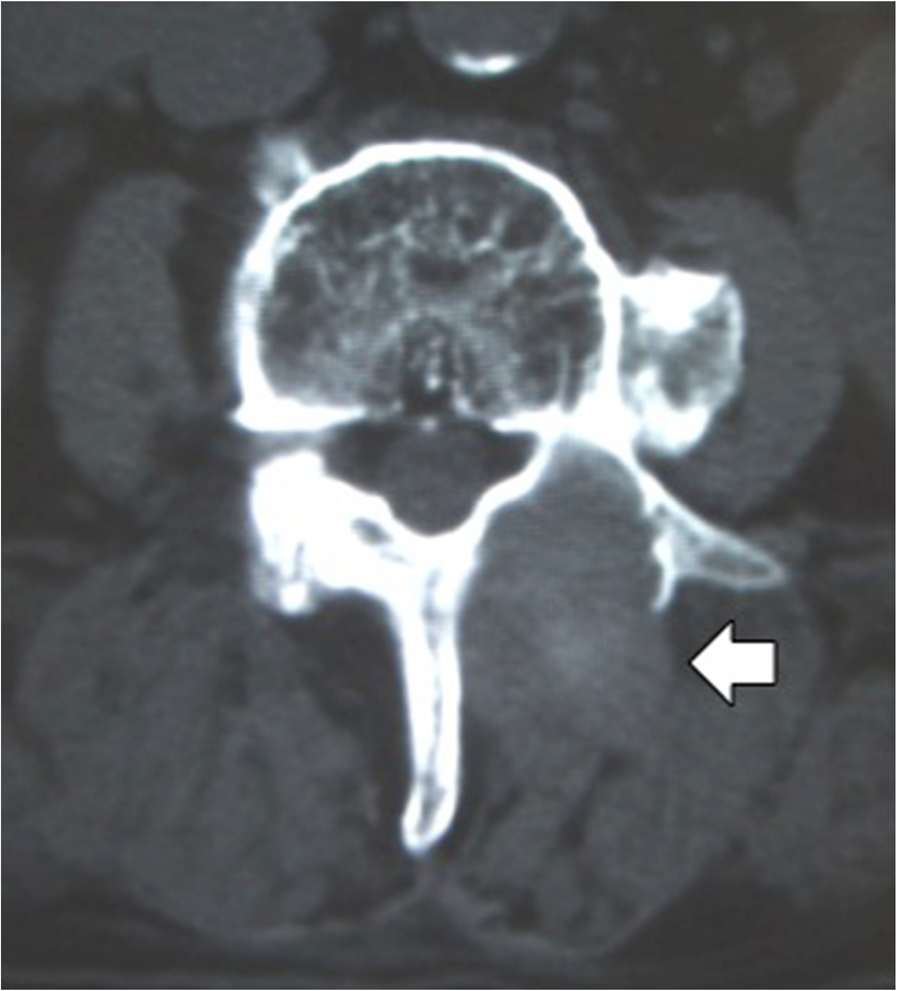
Computed tomography axial image. A mass (open arrow) is seen posterior to the lamina with osteolytic change.
